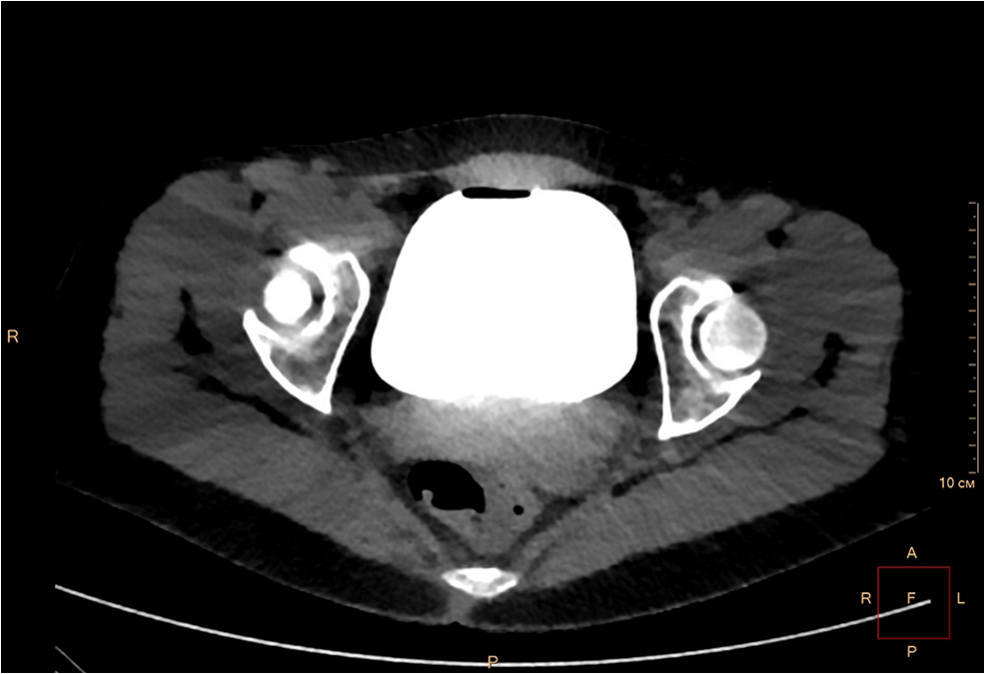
CT cystography, axial plane (retrograde contrast medium instillation). Contrast medium accumulated within the bladder without leakage
